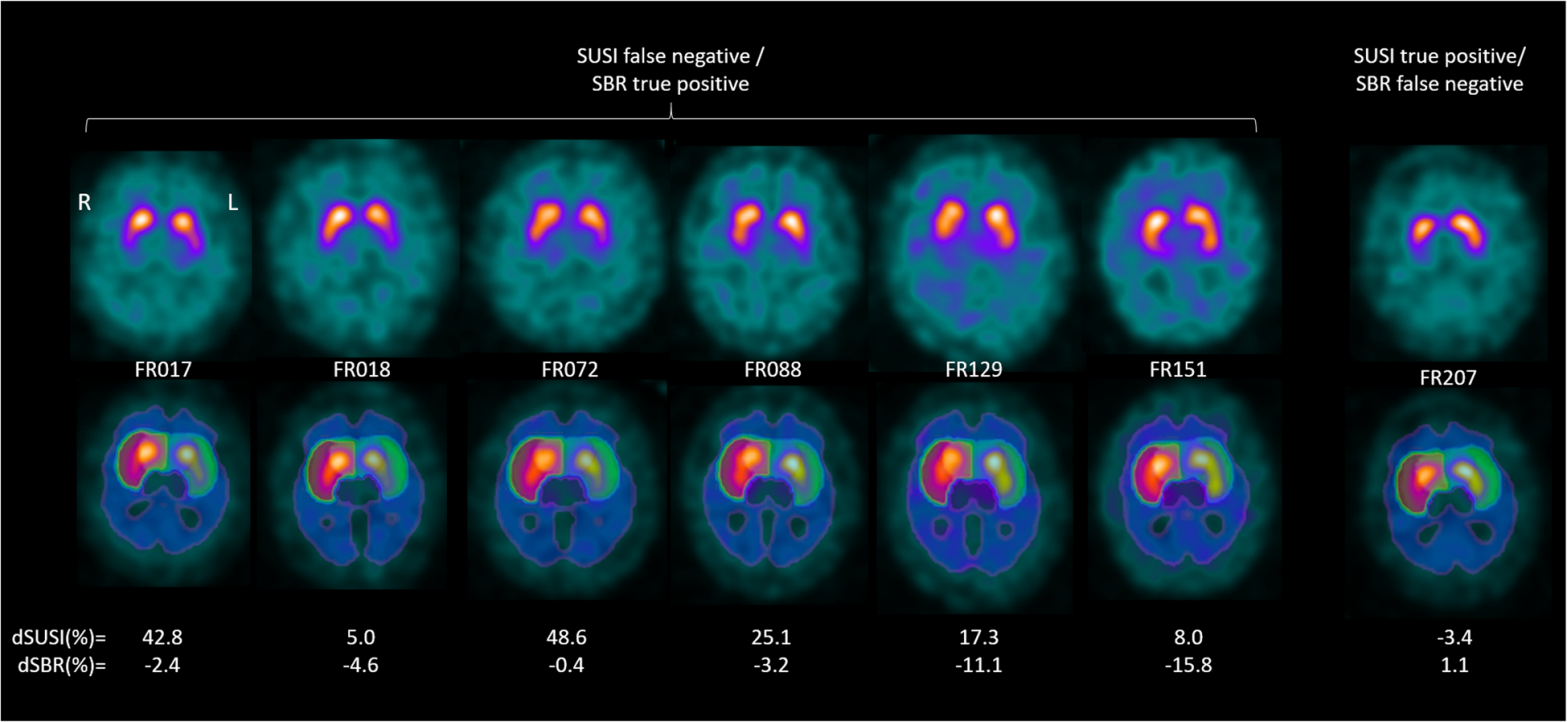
FP-CIT SPECT images from the mono-site/mono-camera setting with discrepant classification as “neurodegenerative PS” or “non-neurodegenerative PS” based on the putaminal SUSI computed in patient space versus classification based on the putaminal SBR. The upper row shows transversal slices through the striatum in patient space. The lower row shows the same transversal slice with the transformed mask of caudate, putamen and the reference region overlaid. dSUSI = 100*(individual SUSI – SUSI cutoff) / SUSI cutoff, dSBR = 100*(individual SBR – SBR cutoff) / SBR cutoff
